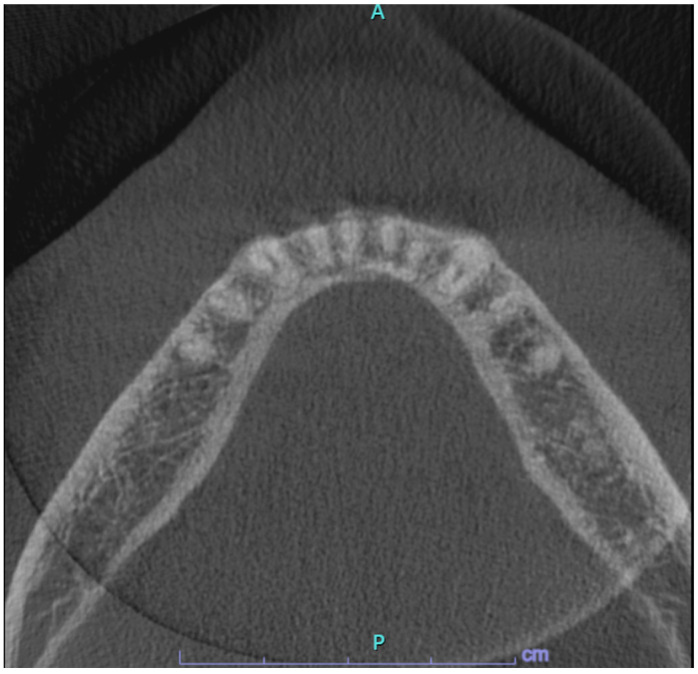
Axial CBCT sections at 2-years follow-up showing the absence of pathological changes.
